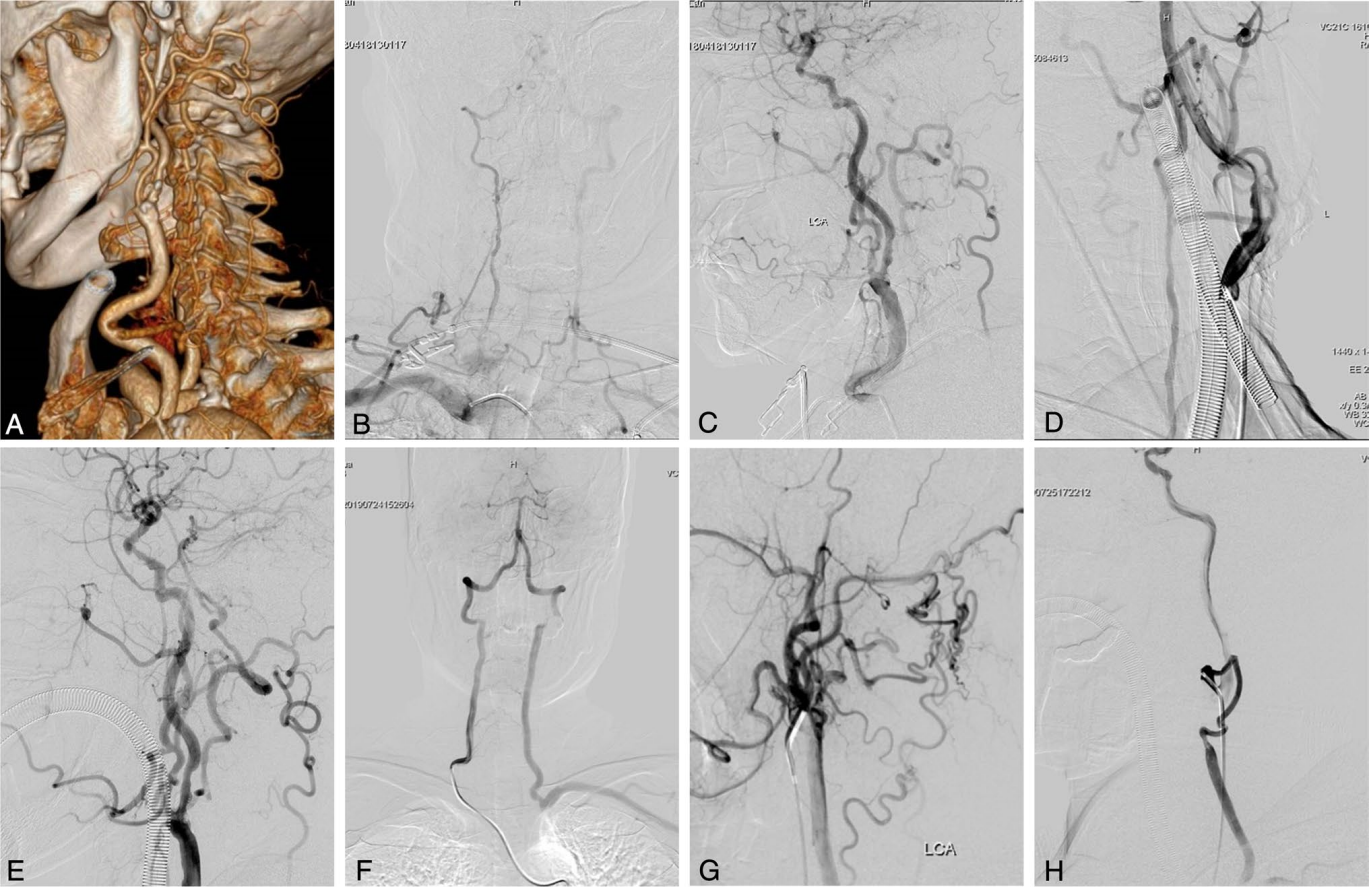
Cases of subclavian steal due to SBA occlusion (Type II). A–E (Case 2): A: CTA shows the occluded left SBA with a residual stump. B: Angiography reveals a hypoplastic contralateral VA with intracranial occlusion and minor steal via intervertebral branches. C: The OA serves as a collateral pathway, though flow is largely diverted through VA and AA/DA steal routes. D: Initial bypass flow is compromised due to persistent steal. E: After VA ligation, steal is markedly reduced, with posterior circulation supply restored. F–H (Case 5): F: Preoperative angiography demonstrates a well-developed right VA contributing pronounced steal; endovascular recanalization failed. G: OA collaterals provide minimal filling to the vertebrobasilar system. H: Without VA ligation, bypass flow participates in the steal phenomenon
